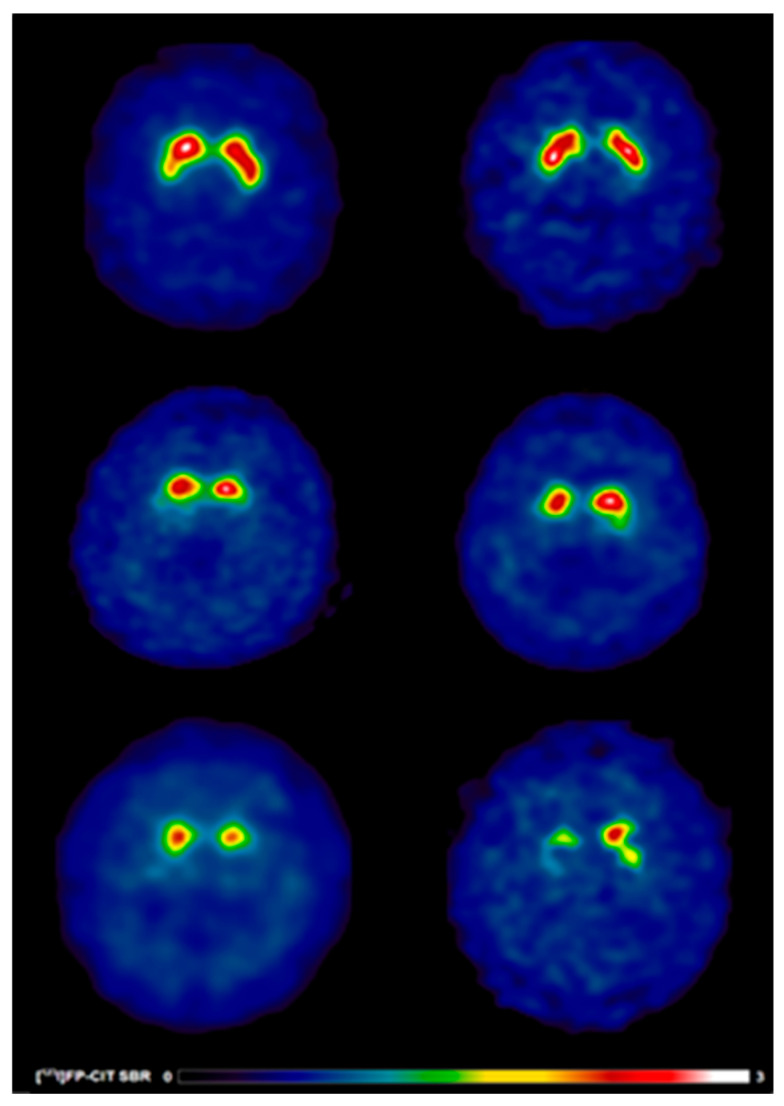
[^123^I]FP-CIT SPECT images in early PD patients with and without speech difficulties. Reduced [^123^I]FP-CIT binding reflects presynaptic nigrostriatal dopaminergic degeneration and should not be interpreted as an index of treatment responsiveness or pharmacological effect. Warmer colours indicate higher dopamine transporter availability, whereas cooler colours indicate reduced presynaptic dopaminergic binding. Anatomical regions of interest include the caudate nucleus and putamen bilaterally. (Top) A 63-year-old healthy control male (left) showing typical [^123^I]FP-CIT specific binding ratios in the caudate (SBR: 3.03) and putamen (SBR: 2.26) and a 69-year-old healthy control female (right) showing typical [^123^I]FP-CIT specific binding ratios in the caudate (SBR: 3.21) and putamen (SBR: 2.79). (Middle) A 63-year-old male (left) without speech difficulties exhibiting slight dopaminergic deficits as reflected by [^123^I]FP-CIT specific binding ratios in the caudate (SBR: 1.98) and putamen (SBR: 0.52) and a 69-year-old female (right) without speech difficulties exhibiting slight dopaminergic deficits as reflected by [^123^I]FP-CIT specific binding ratios in the caudate (SBR: 2.45) and putamen (SBR: 0.79). (Bottom) A 63-year-old male (left) with speech difficulties demonstrating larger striatal dopaminergic deficits as reflected by [^123^I]FP-CIT specific binding ratios in the caudate (SBR: 1.16) and putamen (SBR: 0.37) and a 69-year-old male (right) with speech difficulties demonstrating larger striatal dopaminergic deficits as reflected by [^123^I]FP-CIT specific binding ratios in the caudate (SBR: 1.33) and putamen (SBR: 0.77).
